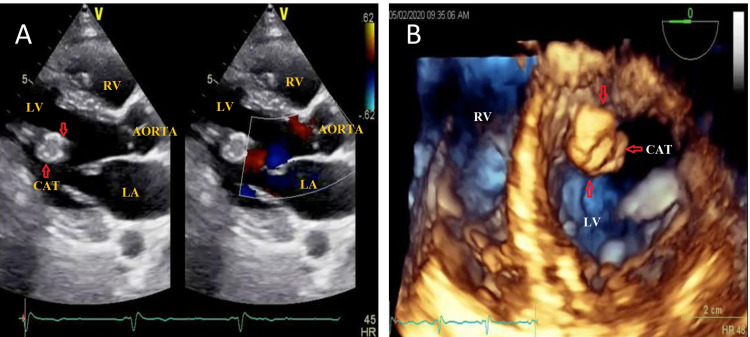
A: Two-dimensional echocardiography, PLAX view showing 1.9 x 1.7 cm pedunculated mass in the LV. B: Three-dimensional echocardiography showing 1.9 x 1.7 cm pedunculated mobile mass in LV attached to interventricular septum. LV, left ventricle; RV, right ventricle; LA, left atrium; CAT, calcified amorphous tumor.
